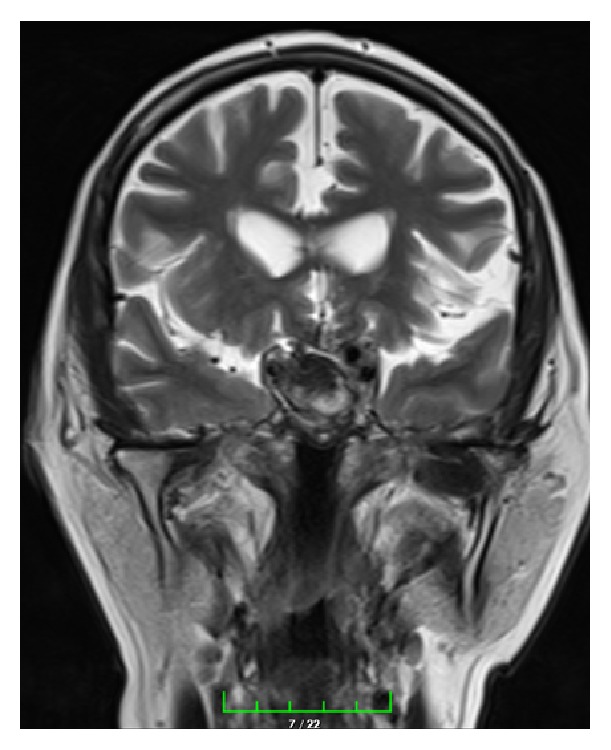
Coronal T2-weighted MR image after embolization.
